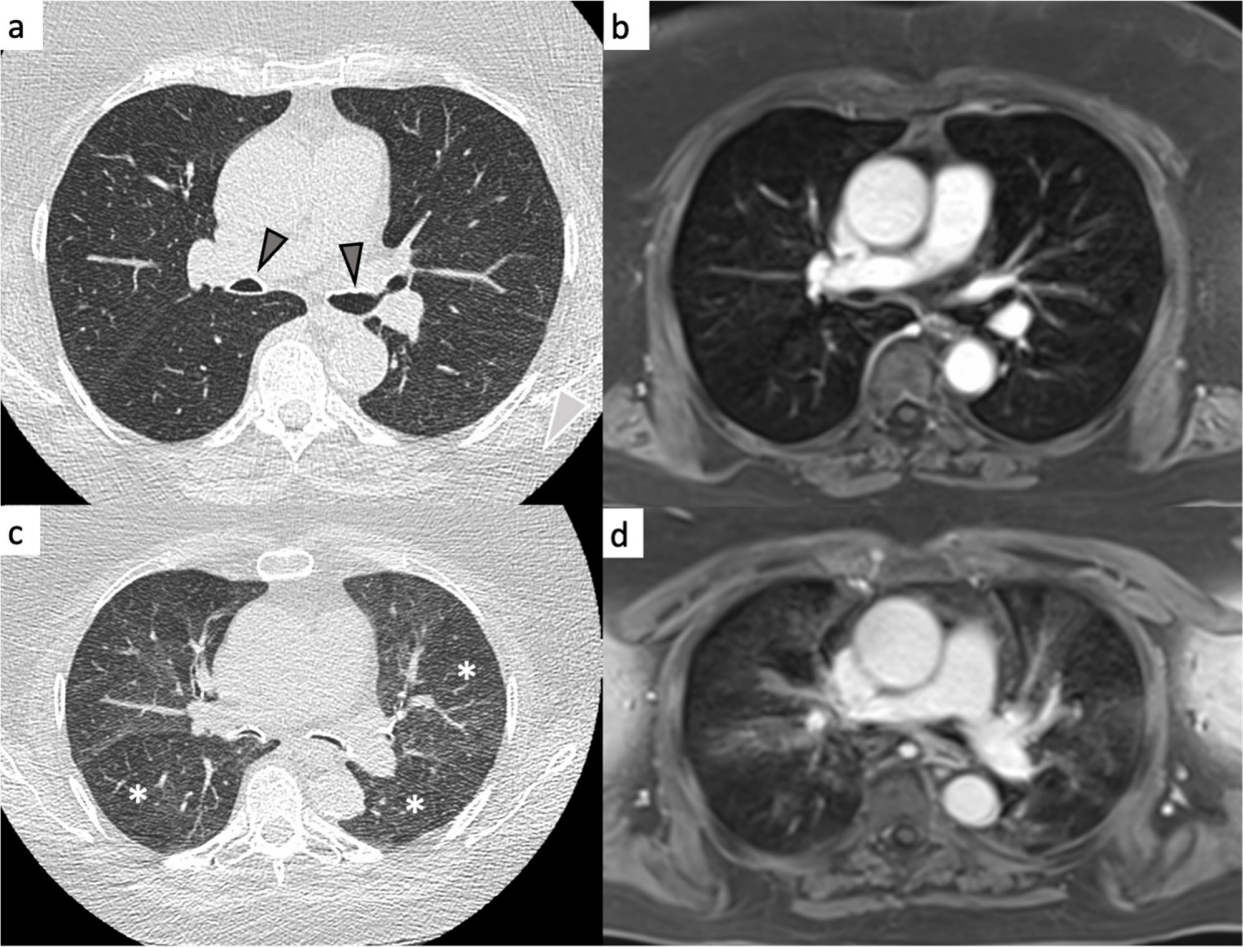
Large and small airway disease in a 70-year-old female participant (classified as airway predominant COPD phenotype). The CT images in in- and expiration (a, c) as well as the MRI in in- and expiration (b, d) show a collapse of the intermediate bronchus and left main bronchus with large areas of air trapping in expiration (asterisks)
